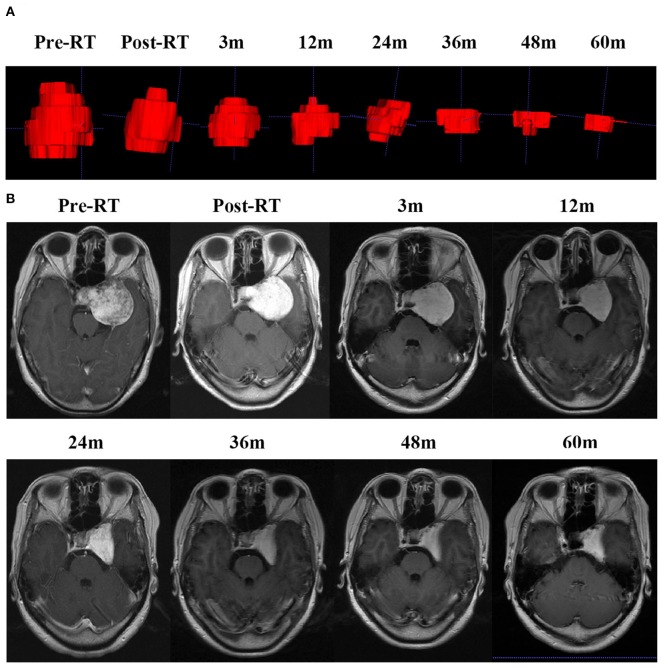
CSH of patient 7 kept decreasing even 5 years later. (A) The 3D imaging of the mass before RT and at different times of follow up after RT. (B) MRI scan before RT, the day after RT completion and at a different time of follow up after RT.
